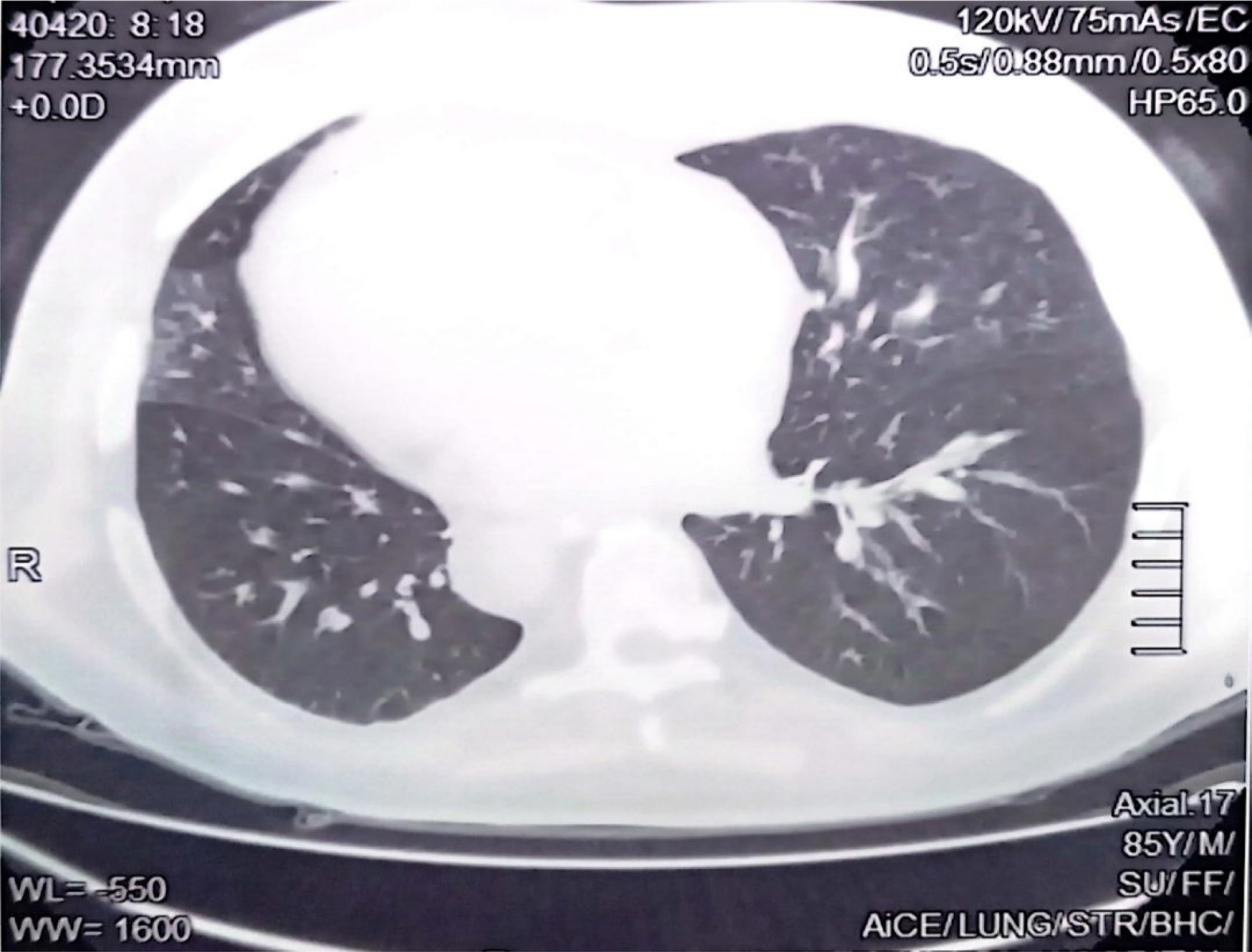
A high‐resolution CT (HRCT) scan of the chest demonstrated focal central bronchiectasis, ground‐glass opacities and pneumatocele.
